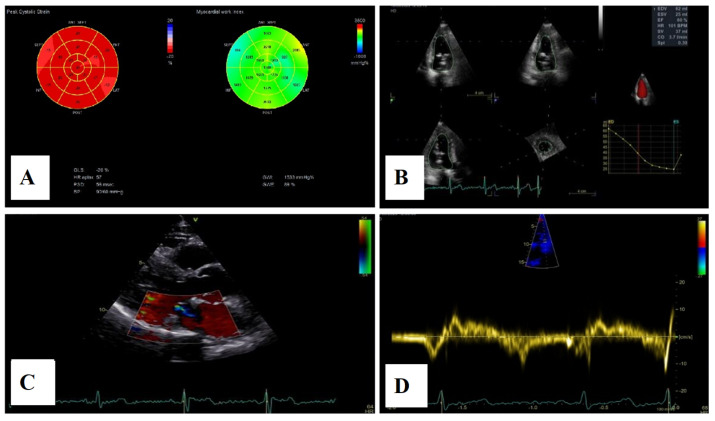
(A)—2D speckle-tracking echocardiography, displaying normal global longitudinal strain (−20%) and myocardial work bull’s-eye plot, displaying normal range of GWI (global work index—1533 mmHg%). (B)—Left ventricular volumetry and systolic function assessed by 3D echocardiography, showing normal volumes and ejection fraction—EF–60%. (C)—The parasternal long-axis view displays trivial mitral regurgitation without mitral annular disjunction. (D)—Tissue Doppler imaging on the lateral mitral annulus, without Pickelhaube sign.
